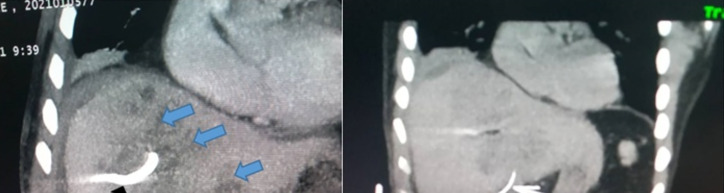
Tomodensitométrie abdominale montrant des abcès hépatiques multifocaux, hypodensité mal limitée (flèches bleues), avec drain transcutané en place (flèche noire) et le corps étranger (flèche jaune) Abdominal computed tomography showing multifocal hepatic abscesses, poorly limited hypodensity (blue arrows), with transcutaneous drain in place (black arrow) and the foreign body (yellow arrow)
